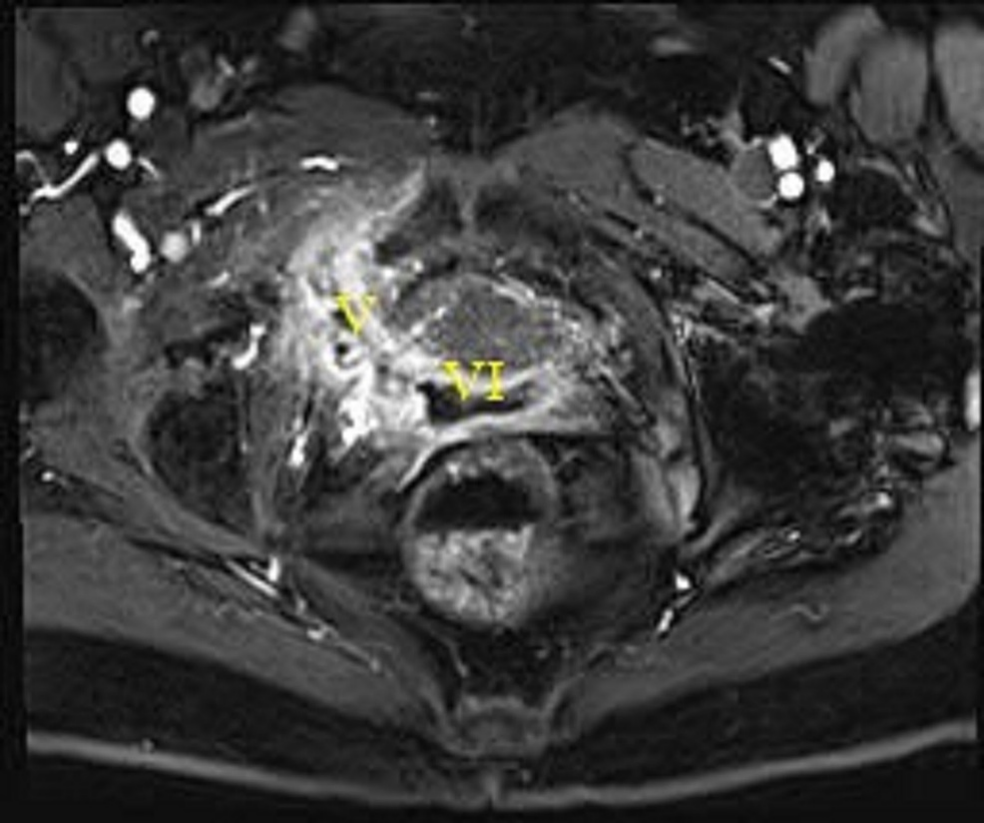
MRI. Fistula tract originating in the right vaginal corner traversing inferior to the pubic ramus V = fistula; VI = vagina
